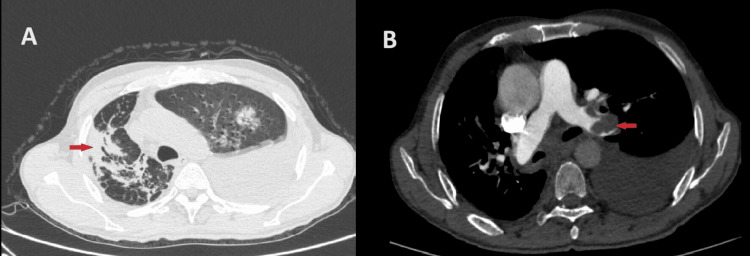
A. High-resolution computerized tomography (HRCT) showing a sub-pleural right-side pulmonary mass lesion of a likely neoplastic etiology with left-side pleural effusion. B. CT-pulmonary angiography (CTPA) showing thrombus in the left main pulmonary artery s/o PTE.
